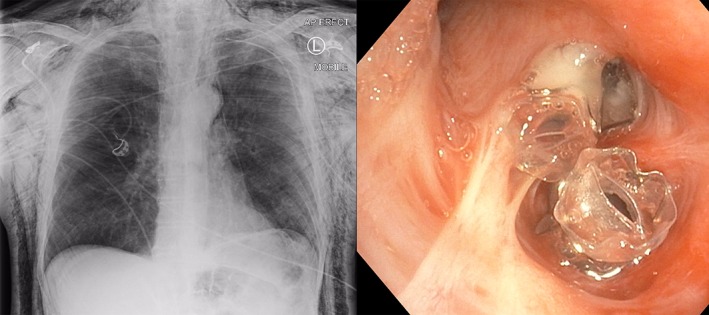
Chest radiograph after thoracostomy tube insertion demonstrating significant subcutaneous emphysema and endobronchial valves in situ in LB3 and LB4+5.
